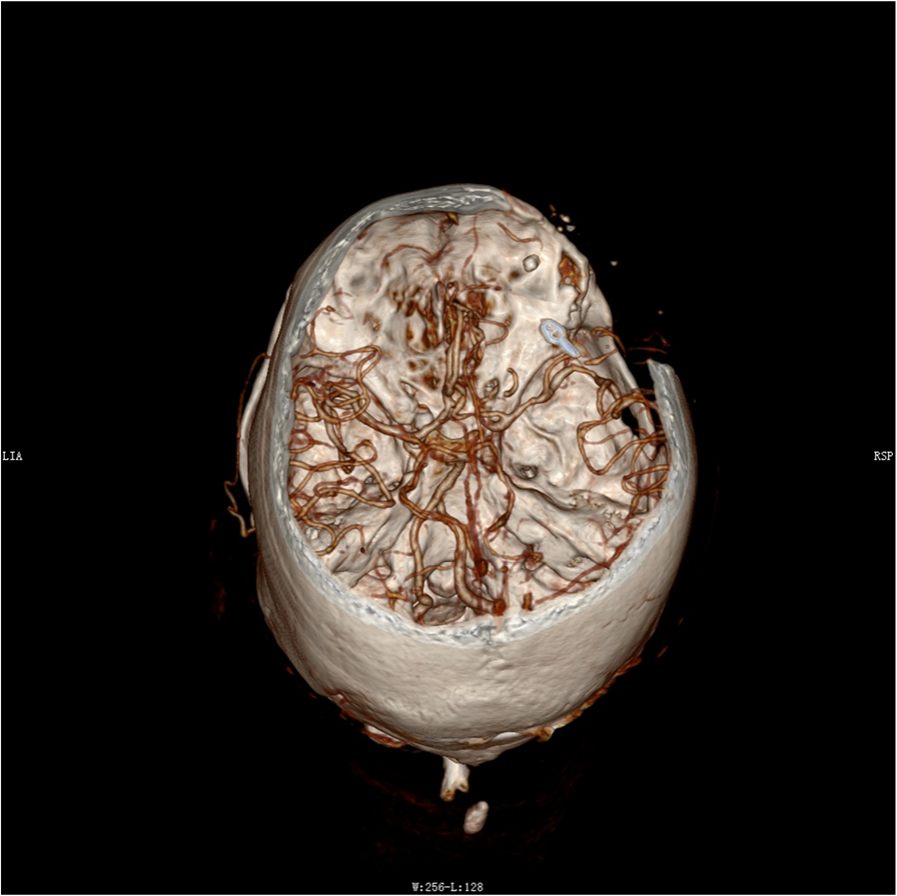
In this three-dimensional computed tomography image, the arrow demonstrates the aneurysm clip at M3 of the right middle cerebral artery. This is an unruptured aneurysm that we found by accident during the first operation. We clipped the aneurysm while exploring the deep foreign body
